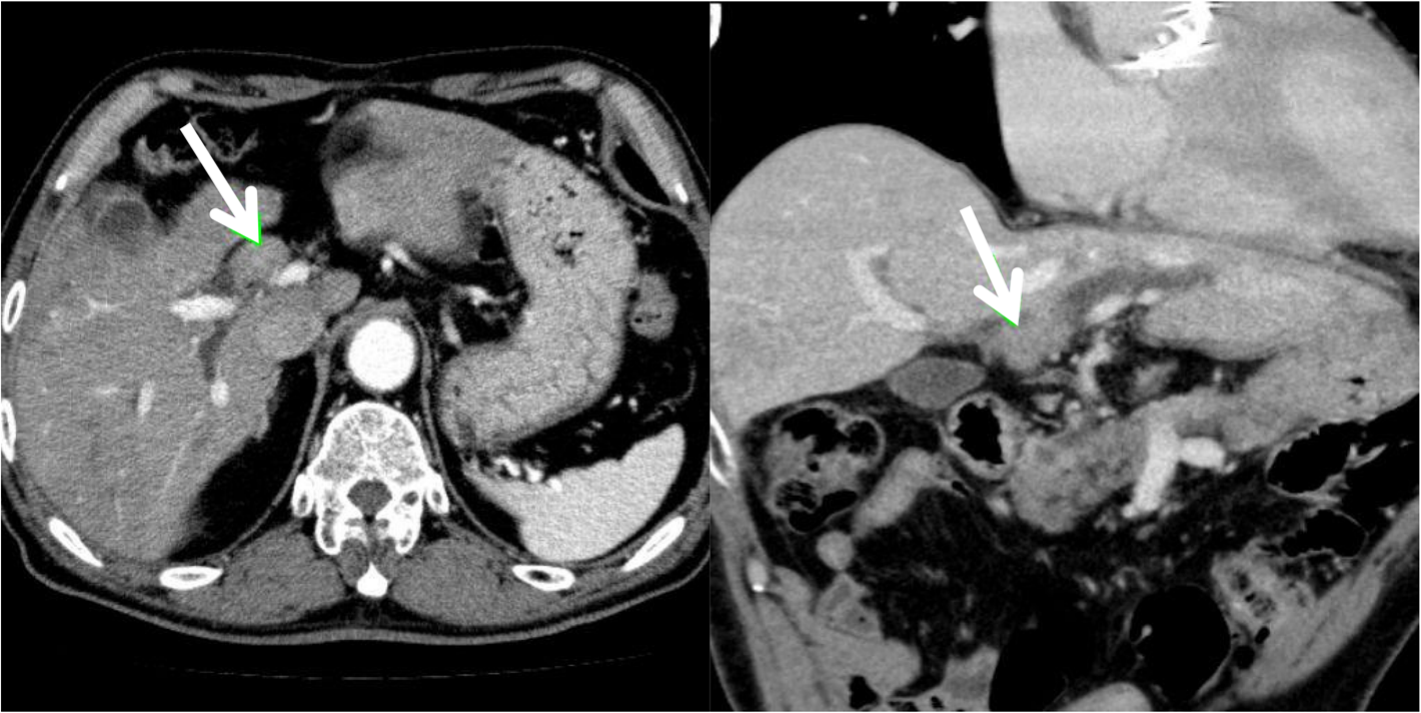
Preoperative enhanced computed tomography. Computed tomography reveals a hilar tumor (*white arrow*) and dilatation of the peripheral bile duct. No space-occupying lesion was detected in the liver parenchyma
